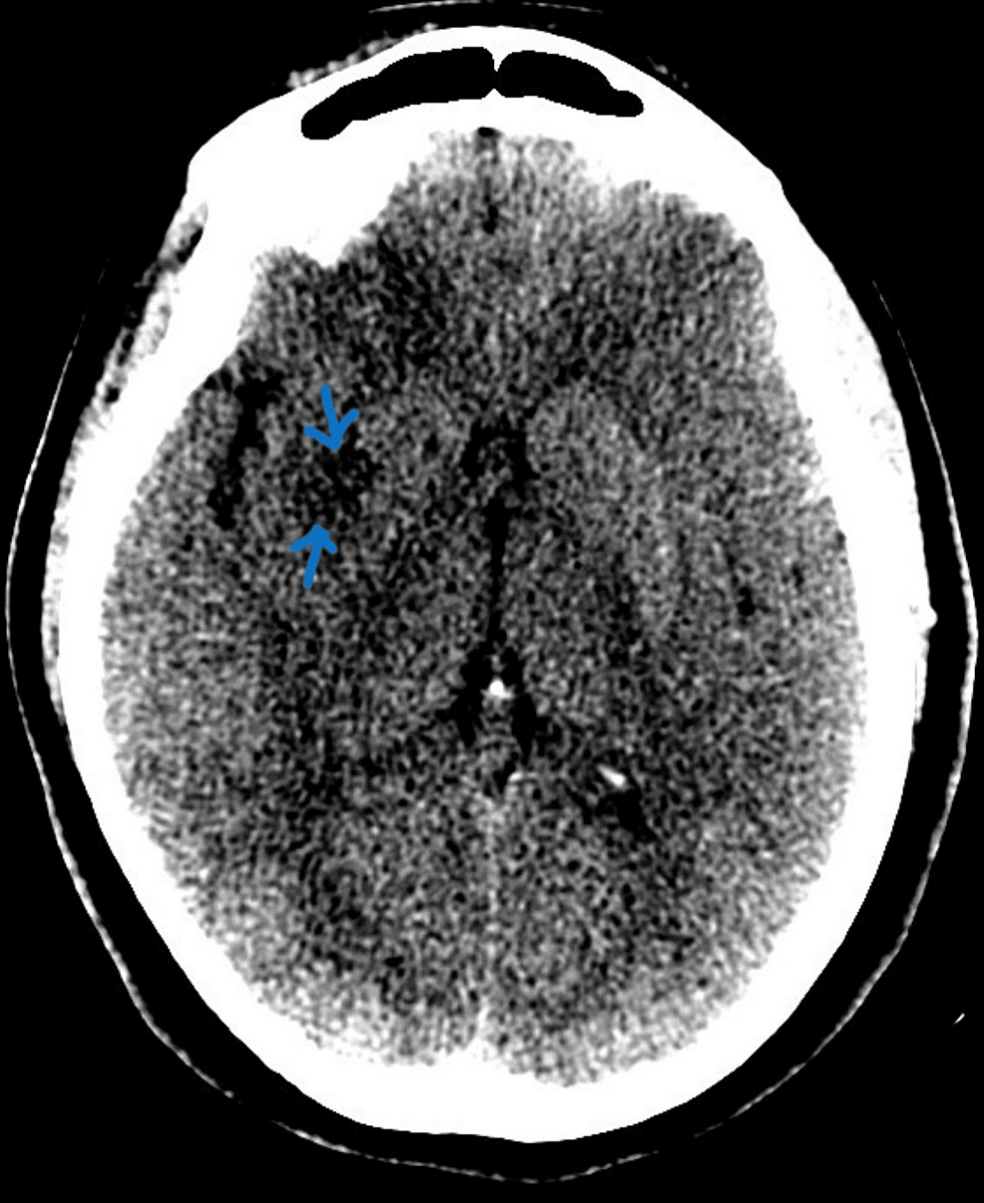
CT scan of the head showing new acute ischemic changes along the right frontal lobe, right corona radiata, and right basal ganglia
